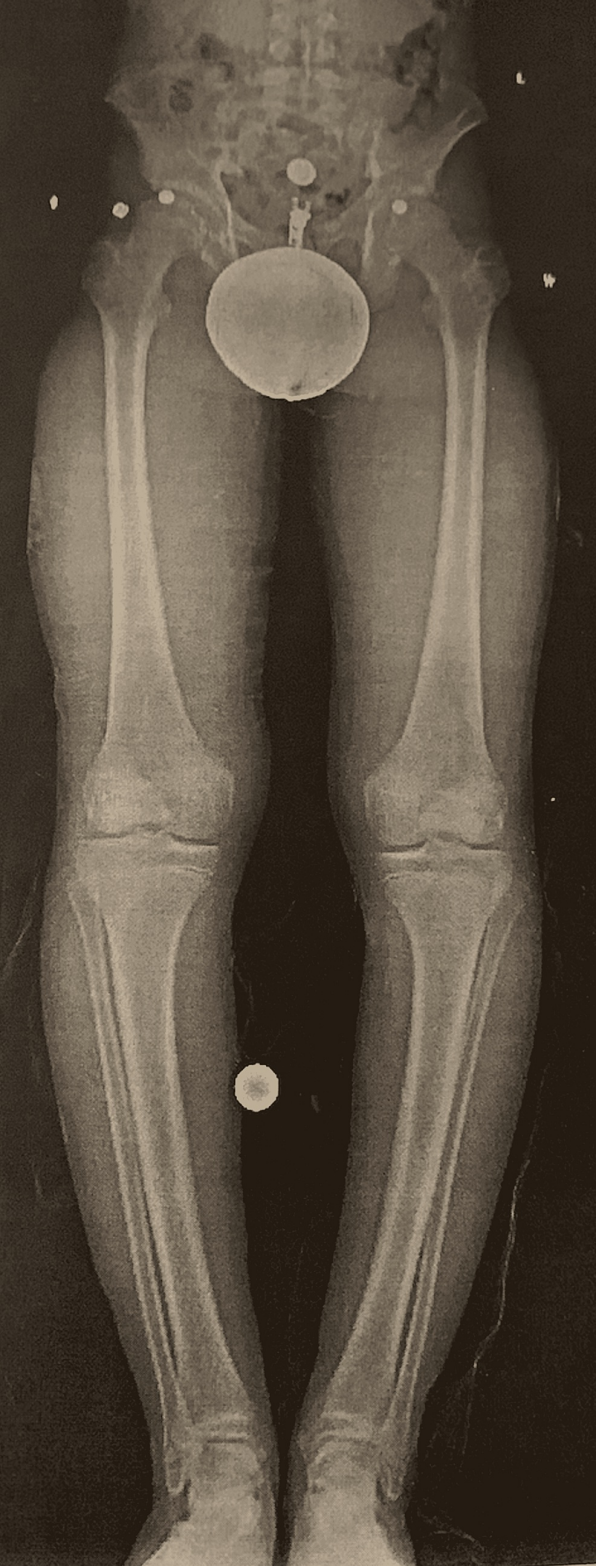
Pre-operative lower extremity X-ray showing the varus deformity bilaterally
